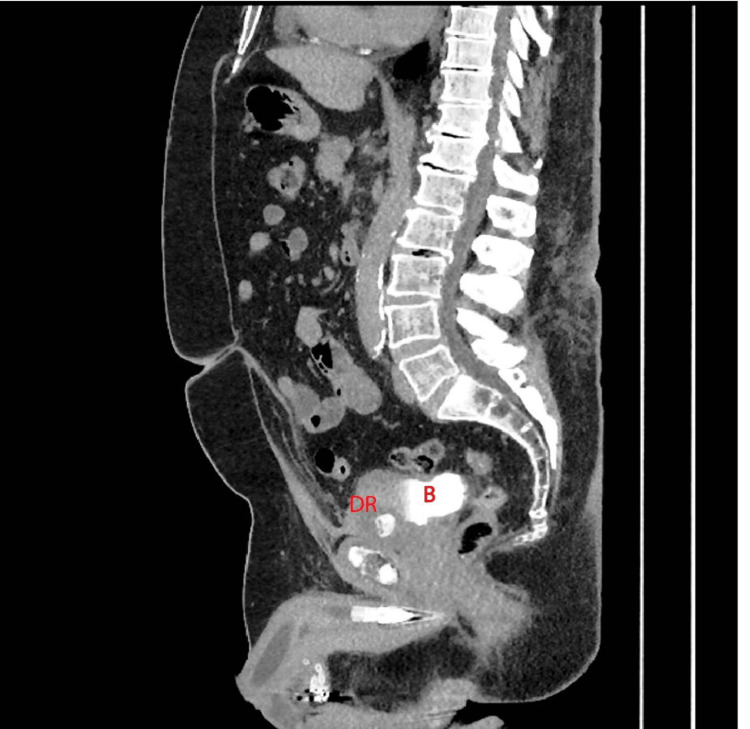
CT urogram longitudinal section showing DR eroding into the bladder B, bladder; DR, decommissioned reservoir
